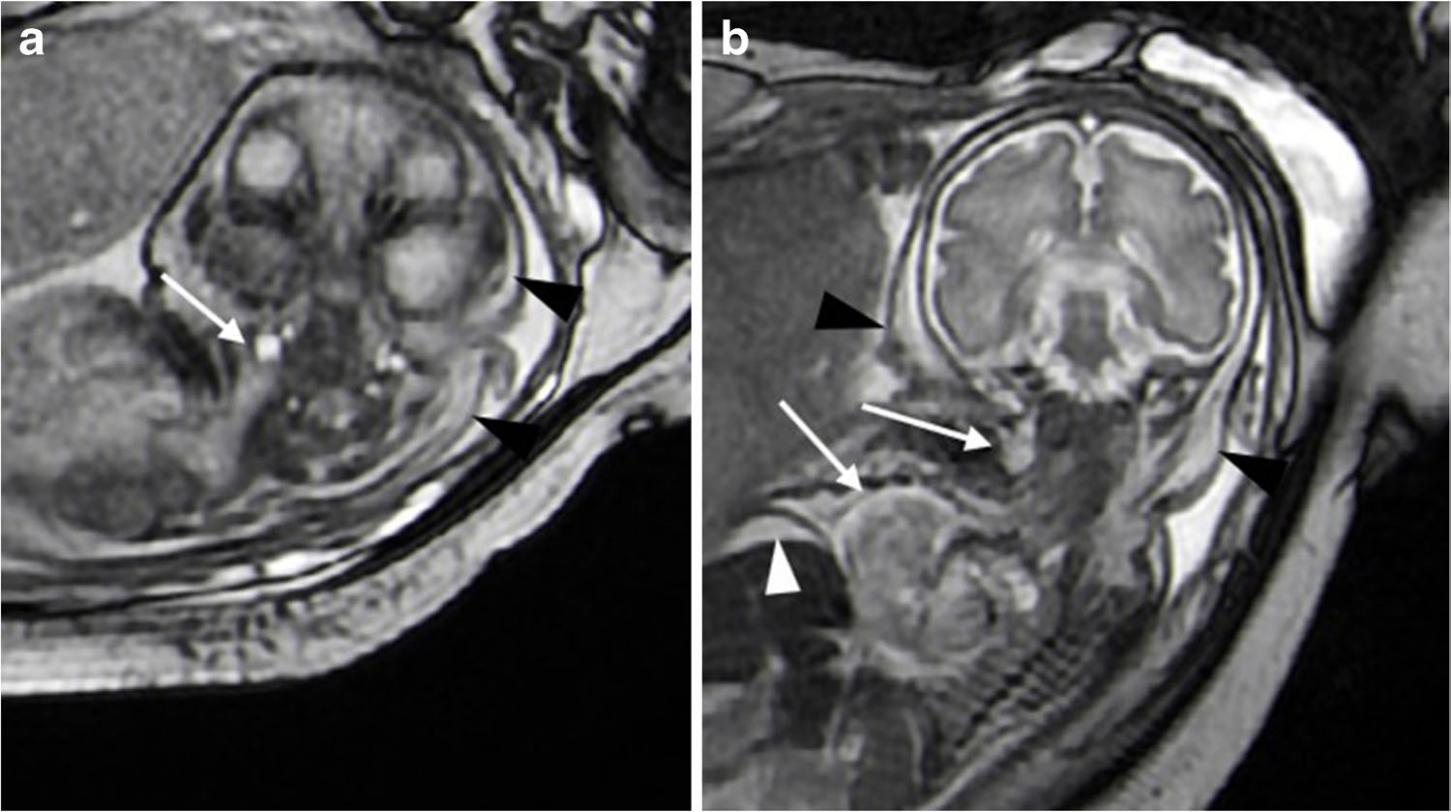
Magnetic resonance imaging in a 30-week-old male fetus with hydrops. a An axial balanced steady-state free precession (B-SSFP) image at the level of the atlantoaxial joint shows fetal scalp oedema (*arrowheads*) and internal jugular vein dilatation (*arrow*), b A coronal B-SSFP image shows fetal scalp thickening due to oedema (*black* *arrowhead*), ascites (*white* *arrowhead*), right atrial enlargement and right internal jugular vein dilatation (*arrows*)
